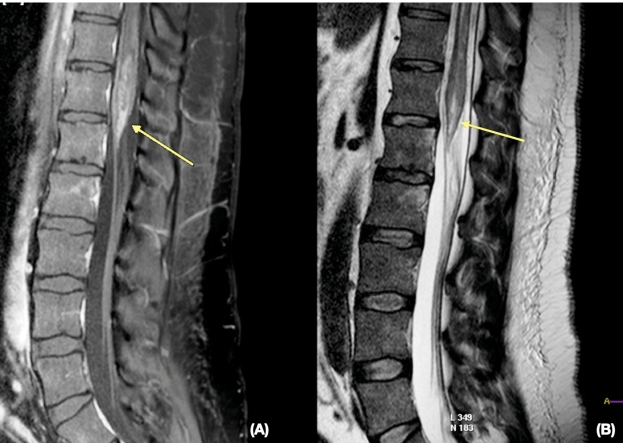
Magnetic resonance imaging of the dorsolumbar spine showing the spinal cord lesion. (A) Sagittal T1-weighted image, after contrast agent administration, showing moderate expansion of the distal spinal cord and conus medullaris with heterogeneous enhancement (arrow). (B) Sagittal T2-weighted image demonstrating a heterogeneous hyperintense lesion (arrow).
